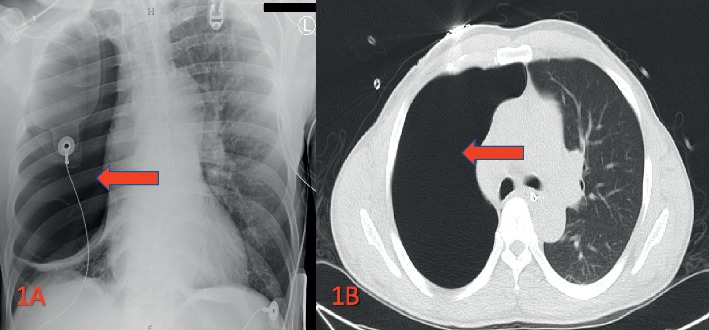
(a) Portable chest X-ray on admission showed extensive lucency in the right hemithorax which suggested large pneumothorax or an air-filled cyst. (b) CT scan of the chest showing one large GBE of the right hemithorax without a mediastinal shift.
